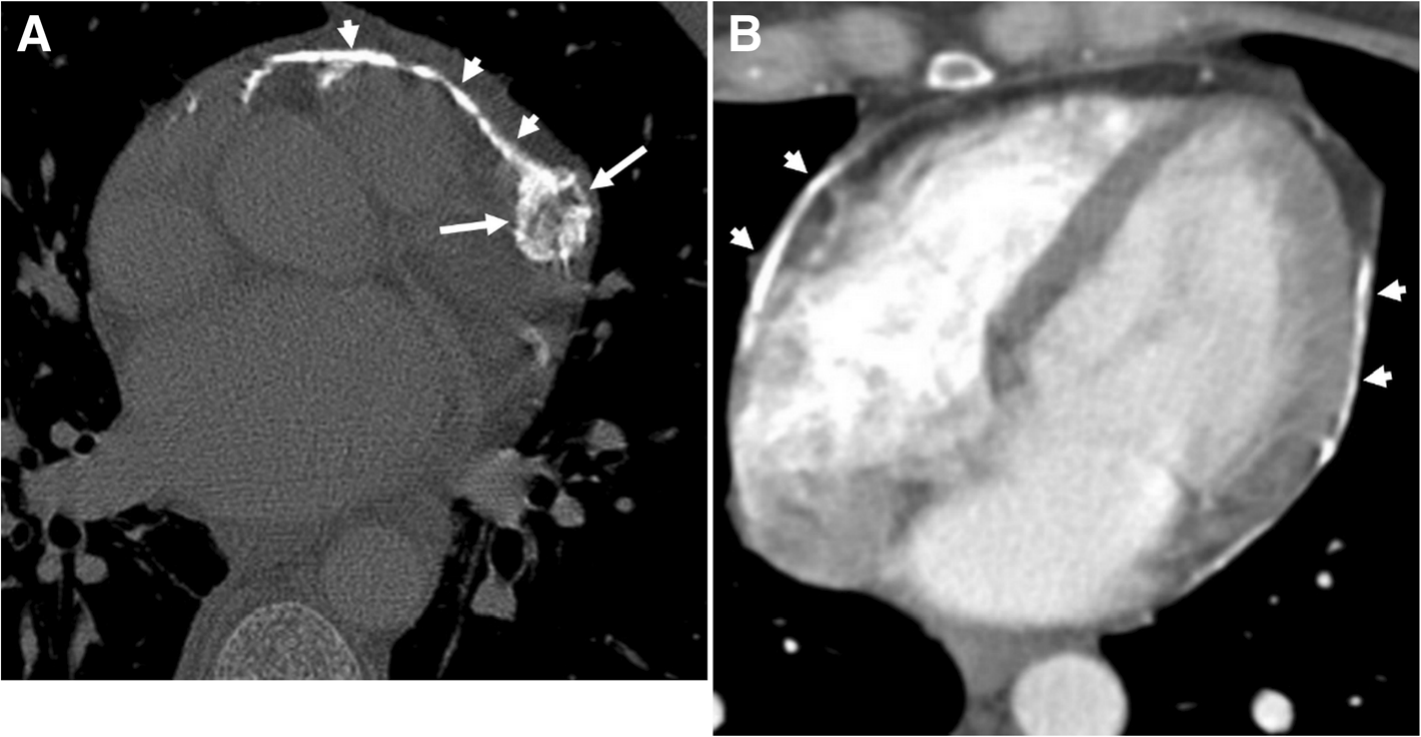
a, b Axial CT images of two different patients demonstrate the nodular (long arrows) and linear (short arrows) areas of calcification through the course of pericardium
